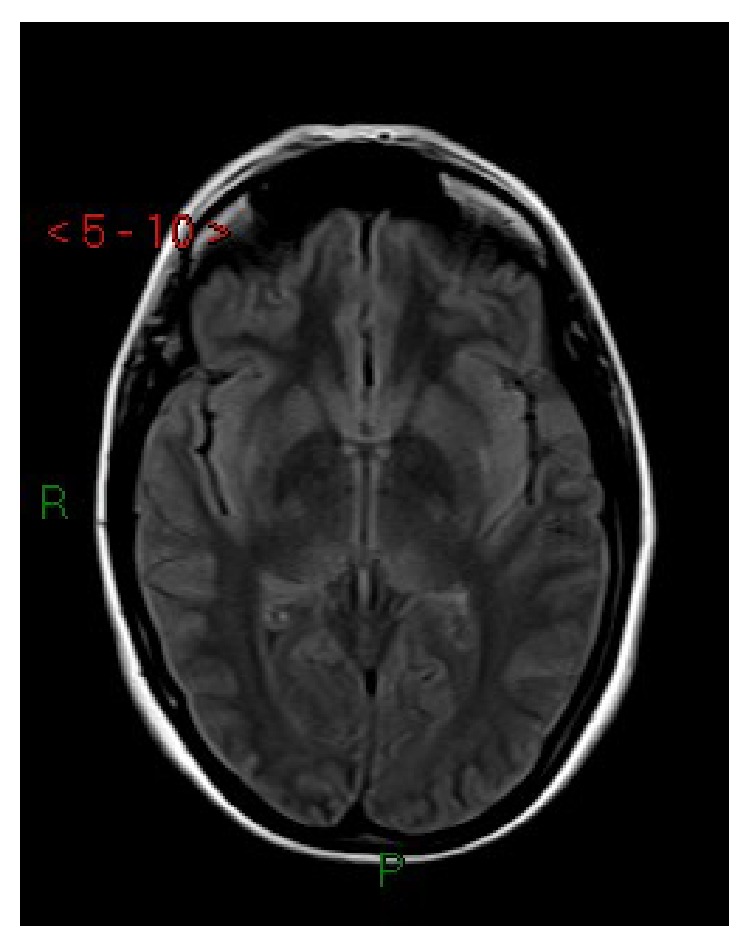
Case 3. Axial T2-FLAIR MRI: cortical-subcortical hyperintense lesions at the insular cortex and bilaterally at the lateral parts of the putamen.
